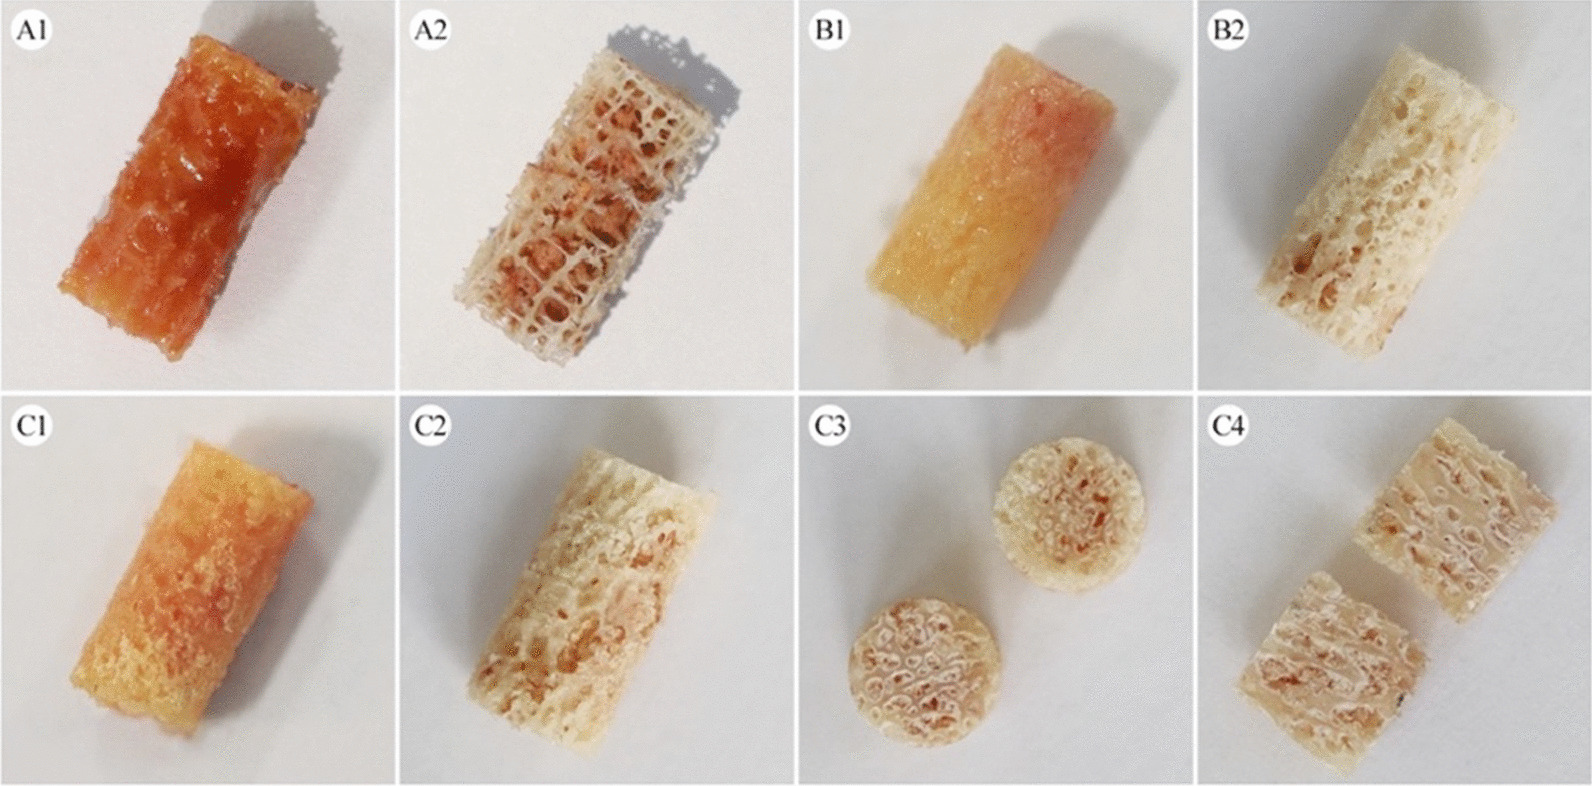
The appearance of non-treated, as well as acetone and saline-treated samples. A1–A2 the low-density sacral sample before and after treatment by a combination of acetone and ultrasonic bath (4 h); B1–B2/C1–C2: the relatively dense femoral head samples before and after treatment by a combination of acetone and ultrasonic bath (4 h); C3–C4: one of the samples (*n* = 3) was split longitudinally and vertically through the center. The samples treated with a combination of ultrasonic bath and acetone (4 h) still had a certain amount of bone marrow left
